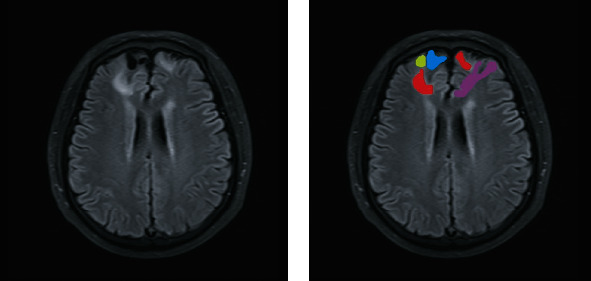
A FLAIR image ROI label example of a 61-year-old man with late epilepsy six months after trauma. Four colors were used: red-labeled FLAIR hyperintense lesion surrounding encephalomalacia; green-labeled FLAIR hypointense lesion surrounding encephalomalacia; blue-labeled encephalomalacia; and purple-labeled contralateral area and adjacent normal part.
